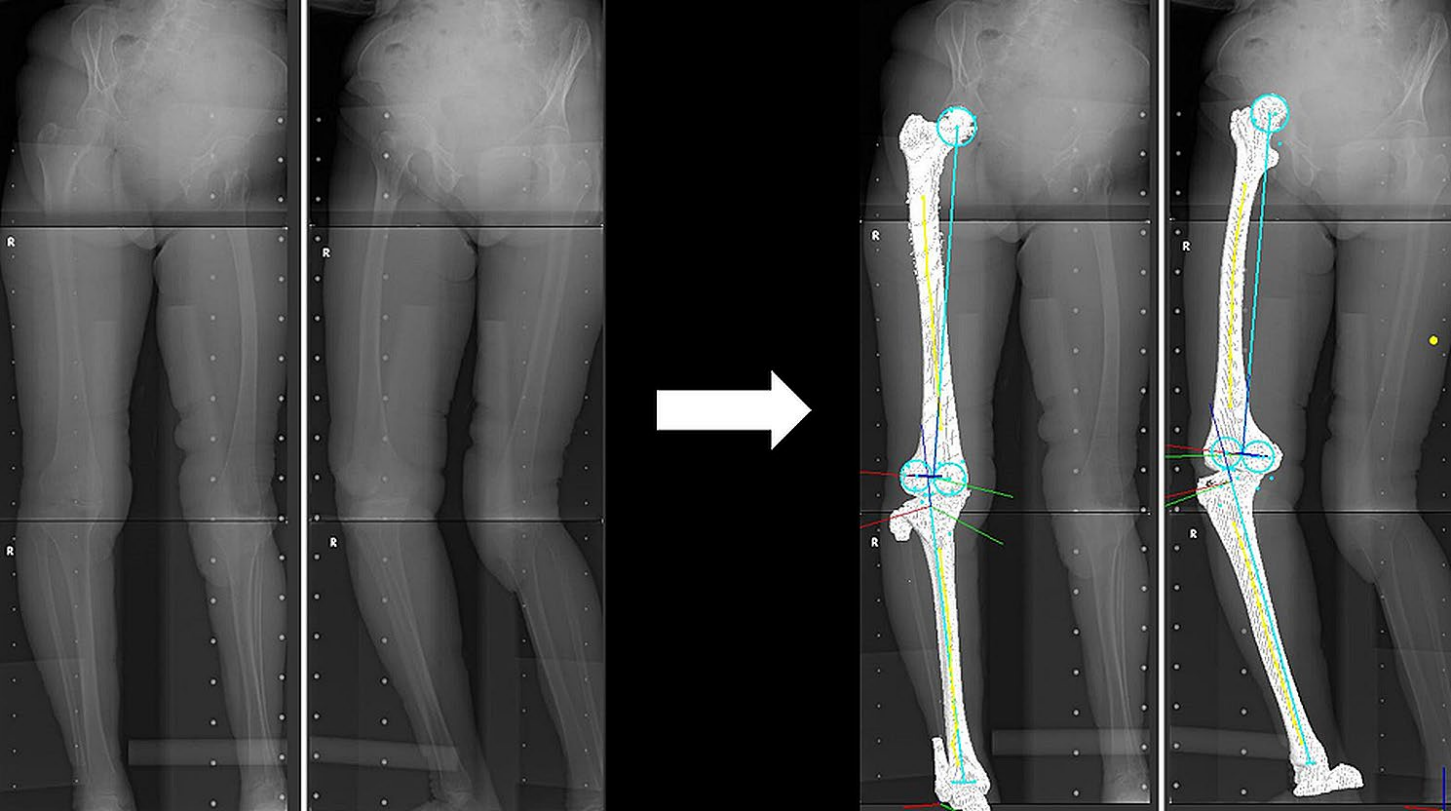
Three-dimensional lower extremity alignment assessment system Thirty-four skin markers were attached to the subjects. For the ten shank markers and 12 thigh markers, the original marker included a steel ball to detect its 2D position on X-ray images. The 3D position of the femorotibial bones can be estimated by superimposing 3D skeletal models onto the bony outline of the lower extremity under weight-bearing conditions using a 3D-to-2D image registration technique
